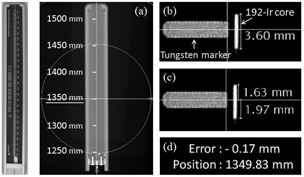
A photograph of the source position check ruler (left) and its d‐FPD images (30.48 cm FOV): (a) the radiographic tungsten markers were placed at 50 mm intervals in the check ruler; (b) definition of the reference length of long axis (3.6 mm) of the ${}^{192}\text{Ir}$ core; (c) measurement of the distance from the intersection between the midline of the tungsten marker and a ${}^{192}\text{Ir}$ core to both ends of ${}^{192}\text{Ir}$ core; (d) the ${}^{192}\text{Ir}$ source positional error was calculated as the difference from 1.8 mm.
